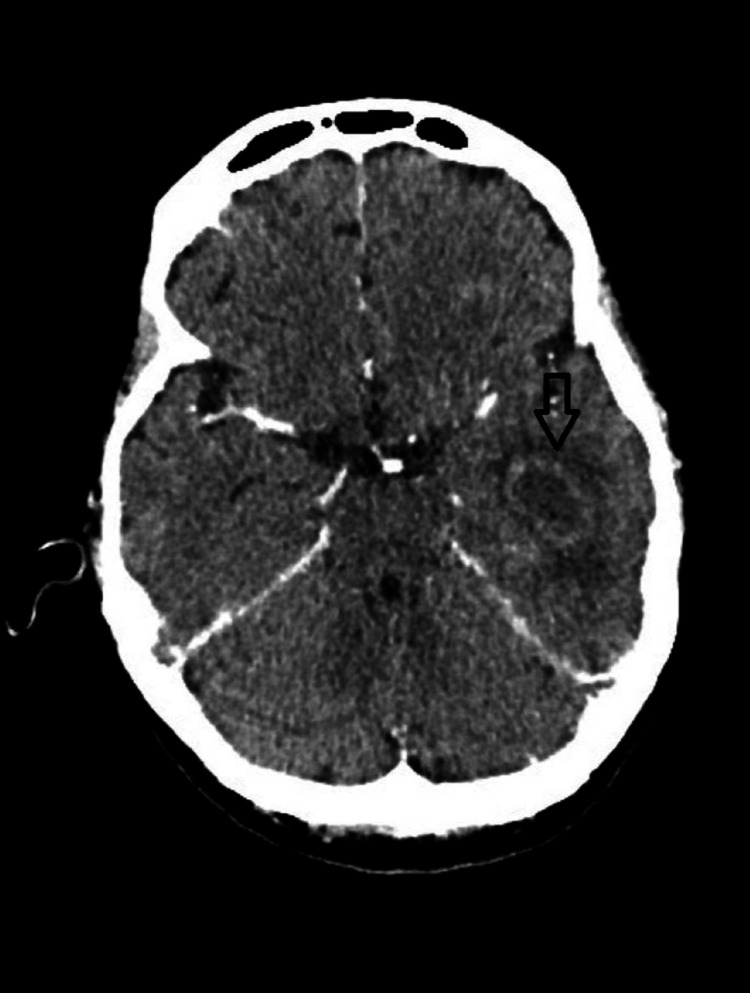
A CT scan of the brain with contrast medium showing a 3 x 1.7 cm ring-enhancing abscess in the left temporal lobe
